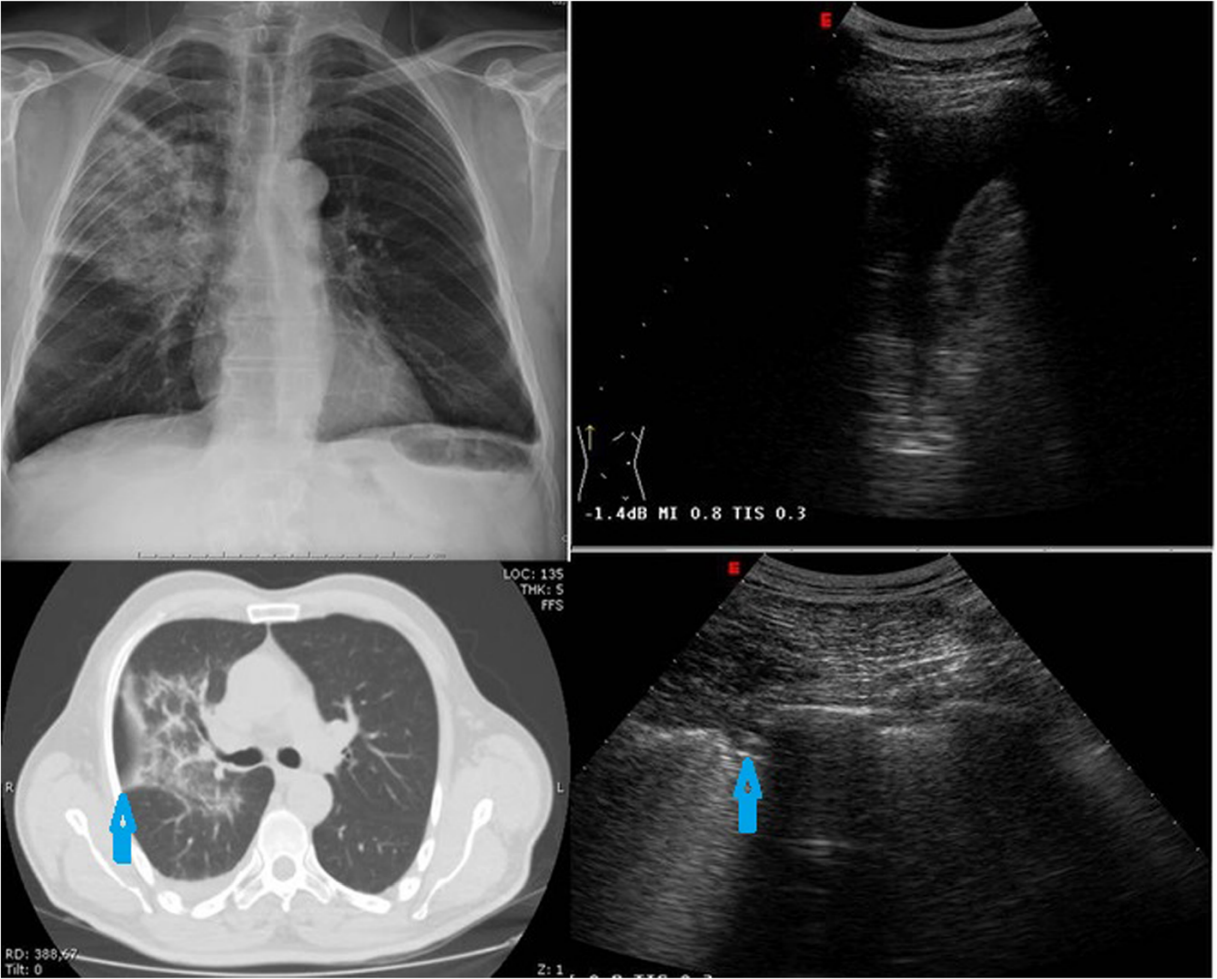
Right lobar pneumonia. Lung consolidation is well defined by CXR (top left) and CT (bottom left); by TUS, pleural effusion is easily identified (top right) but only a very small density and adherent to pleura pneumonia is visible (blue arrows) (bottom right)
